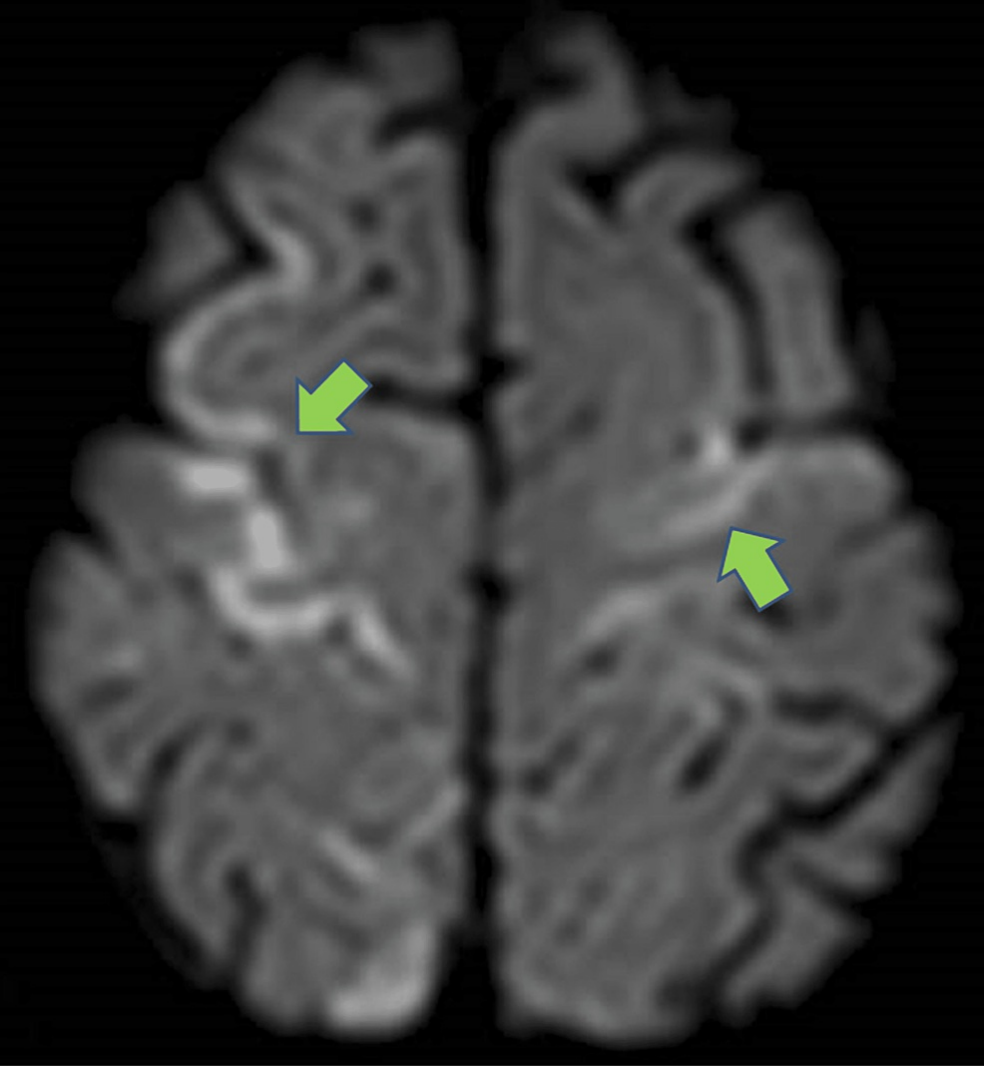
Magnetic resonance imaging (MRI) brain diffusion-weighted imaging (DWI) sequence showing gyriform foci of restricted diffusion in keeping with acute watershed infarcts as a result of earlier cerebral air emboli.
